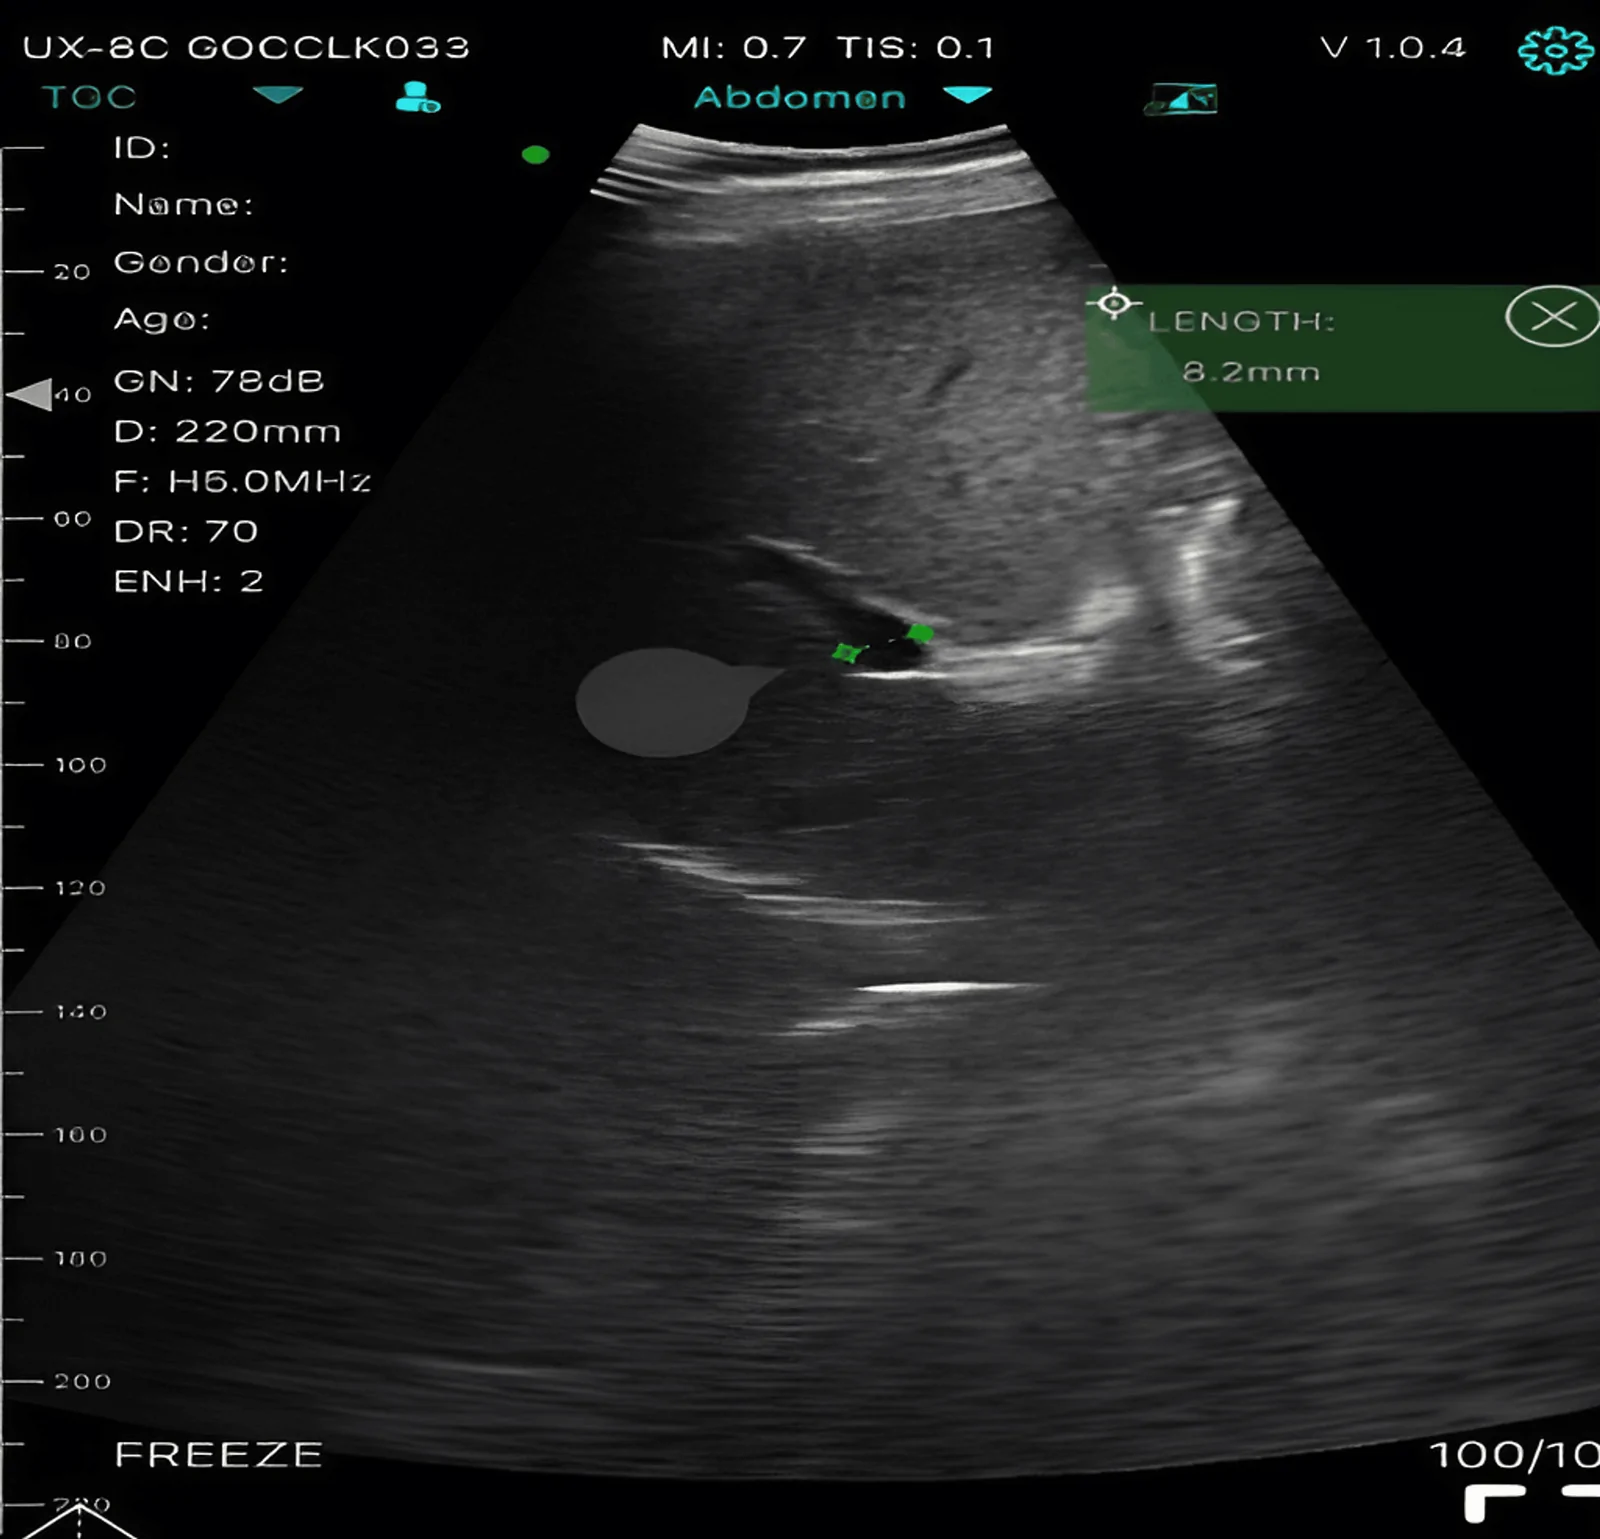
Assessment of the portal vein diameter by wireless handheld ultrasound
